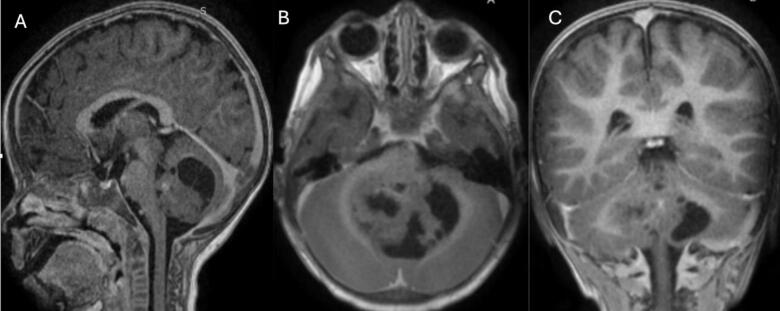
Magnetic resonance imaging of the brain: First MRI done before the first.
